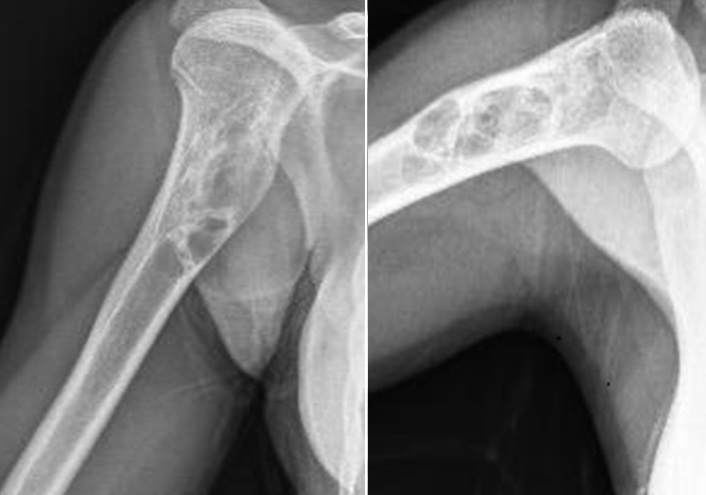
Radiography view of the tumour recurrence in one year after surgery
